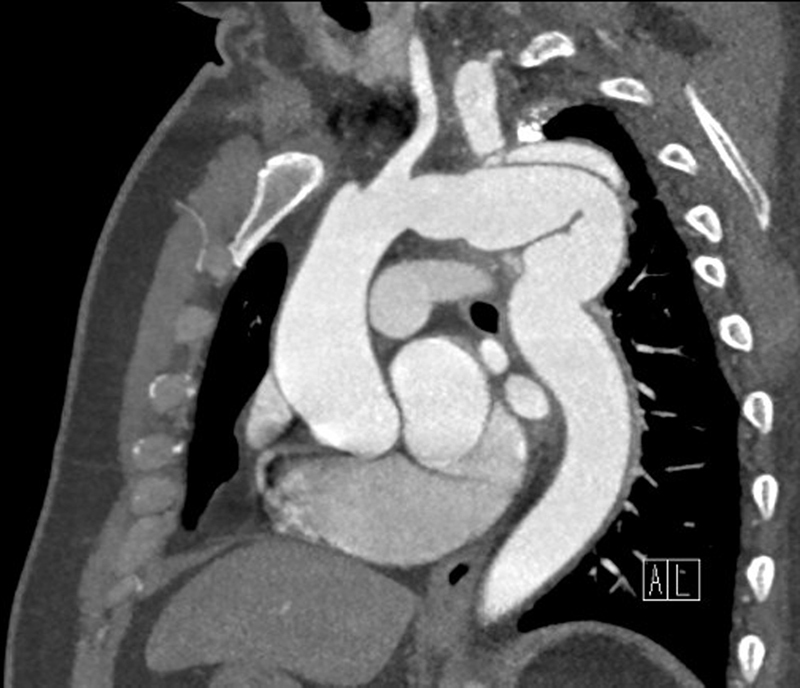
Repeat computed tomography scan confirming successful repair.
